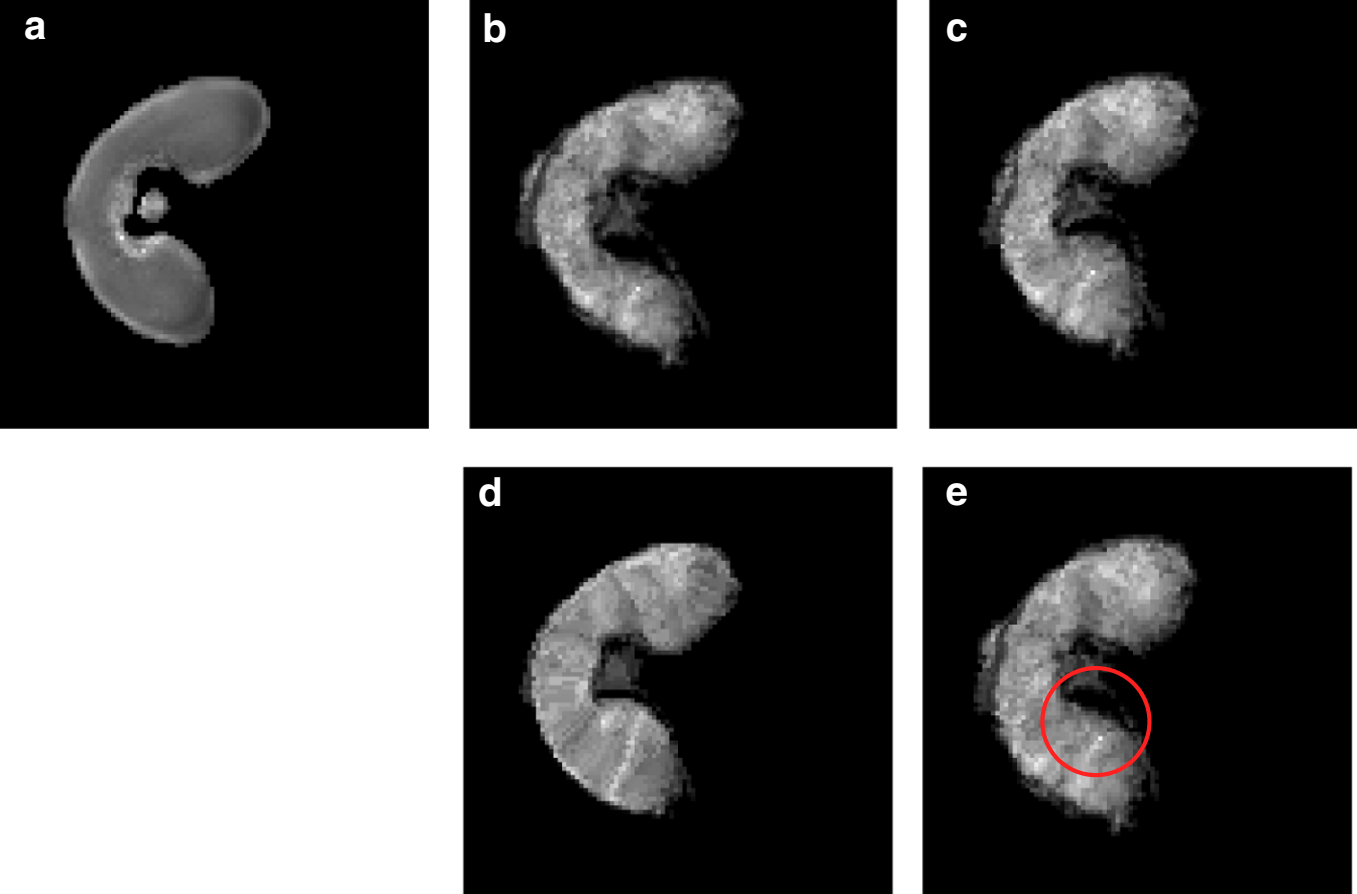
Registration of the MALDI–MSI image onto the MRI image. Registration of the segmented MALDI–MS image onto the a denoised MRI image: b linear registration followed by c the variational method of Modersitzki [21] or d, e free form deformation models with two different parameters ($$\text {FFD}_1$$ and $$\text {FFD}_2$$). e The circled area (in red) shows a difference in shape compared to the MRI image
